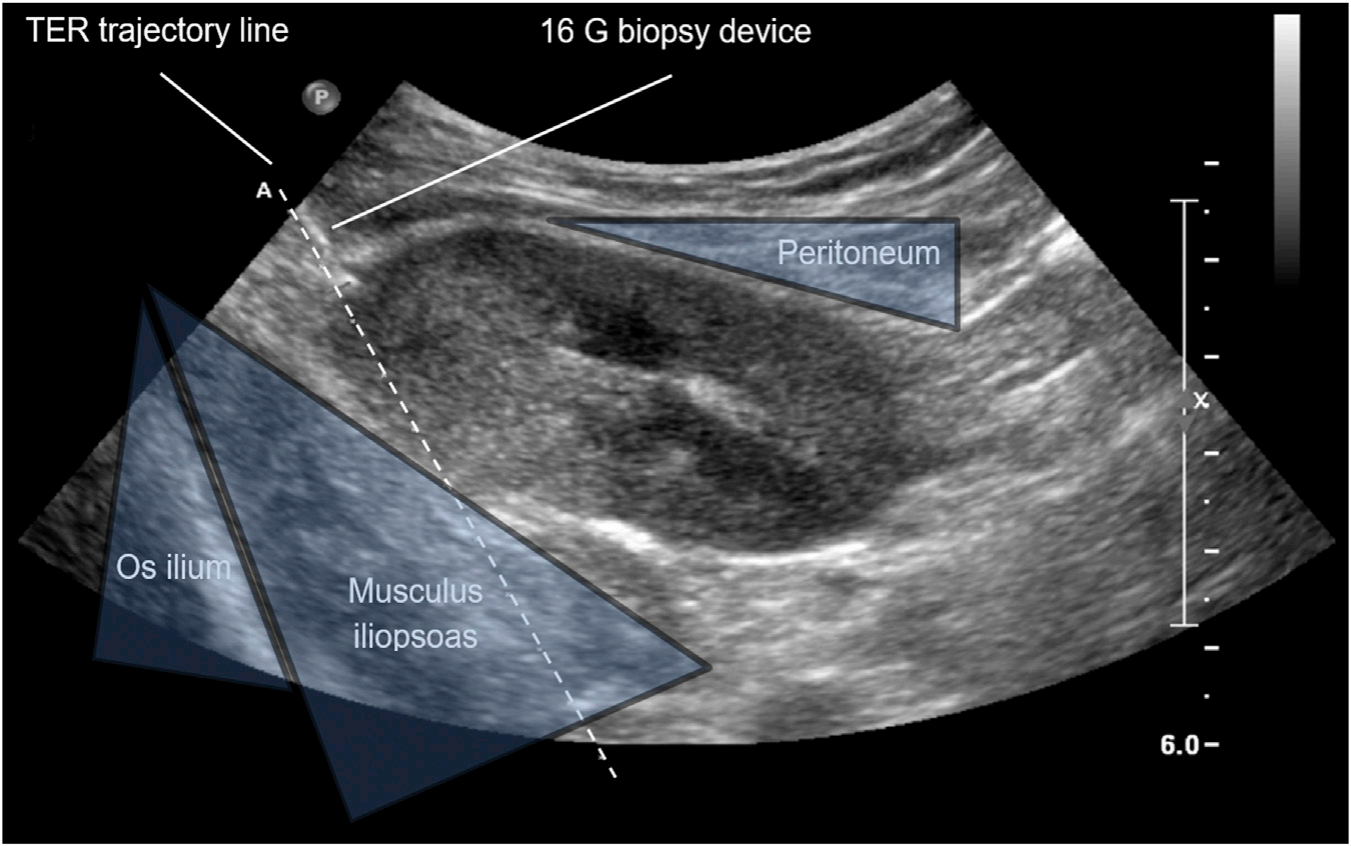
Anatomic landmarks of real-time ultrasound-guided kidney allograft biopsy. Ultrasound image of the right iliac kidney allograft; TER, tangential, extraperitoneal, retrorenal; G, gauge.
